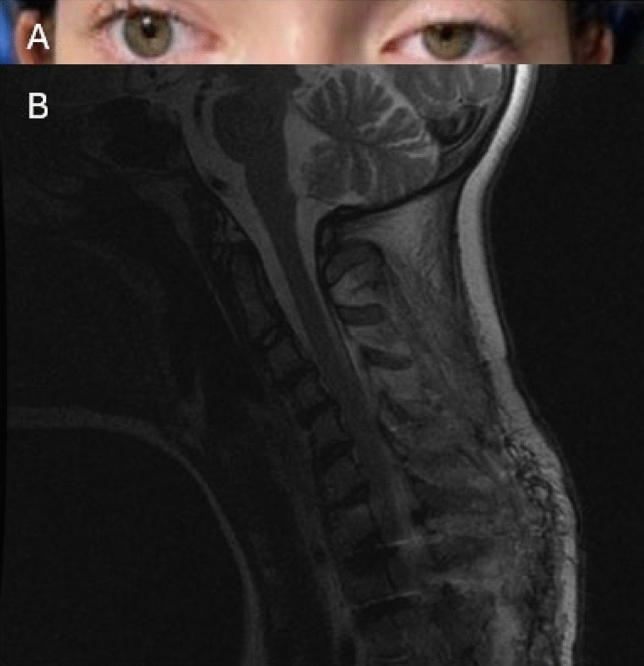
(A) Case 1 Clinical manifestation of ptosis and miosis; (B) MRI cervical spine lateral of a 12-year-old female with DiGeorge syndrome and syndromic scoliosis
